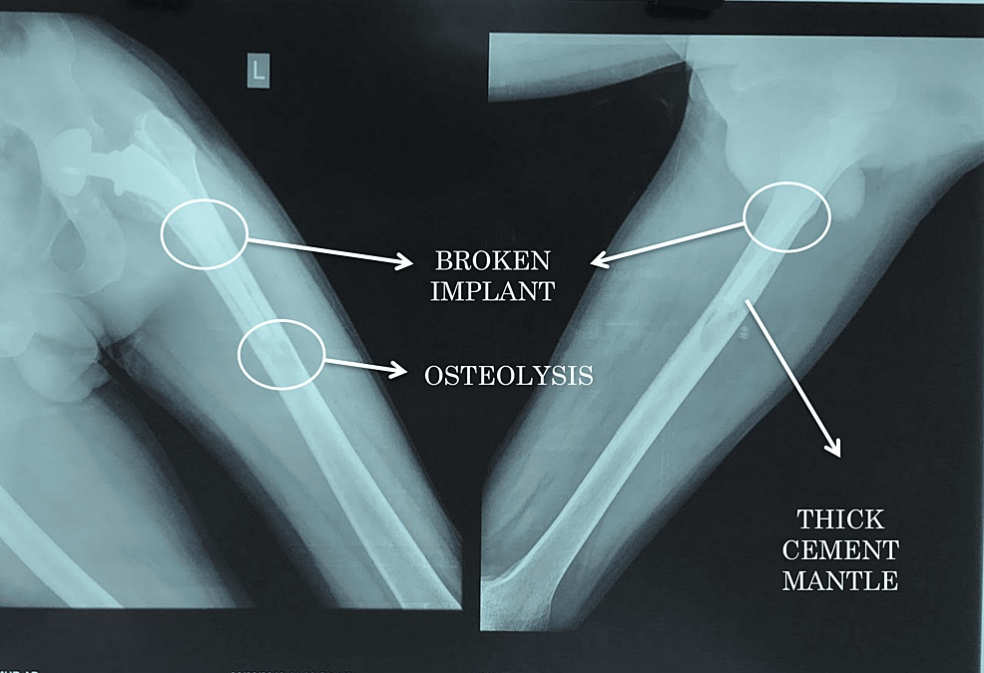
Plain radiograph of the left femur showing the broken implant, osteolysis, and thick cement mantle
